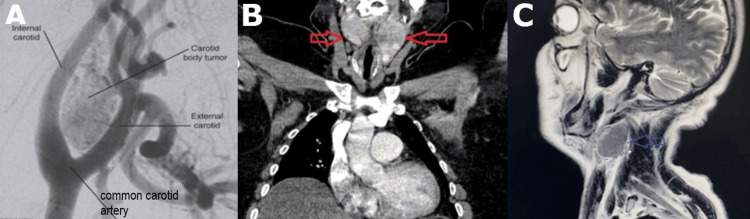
Radiologic diagnosis A) Angiographic view of CBT localized in carotid artery bifurcation; Rutherford's Vascular Surgery and Endovascular Therapy, 9th Edition, chapter 95. B) CT angiographic view of bilateral CBT (red arrows). Image Credits: Yasin kılıç; C) The salt and pepper appearance of CBT on MRI (blue arrow). Image credits: Izatullah Jalalzai. CBT, carotid body tumor; MRI, magnetic resonance imaging; CT, computed tomography
